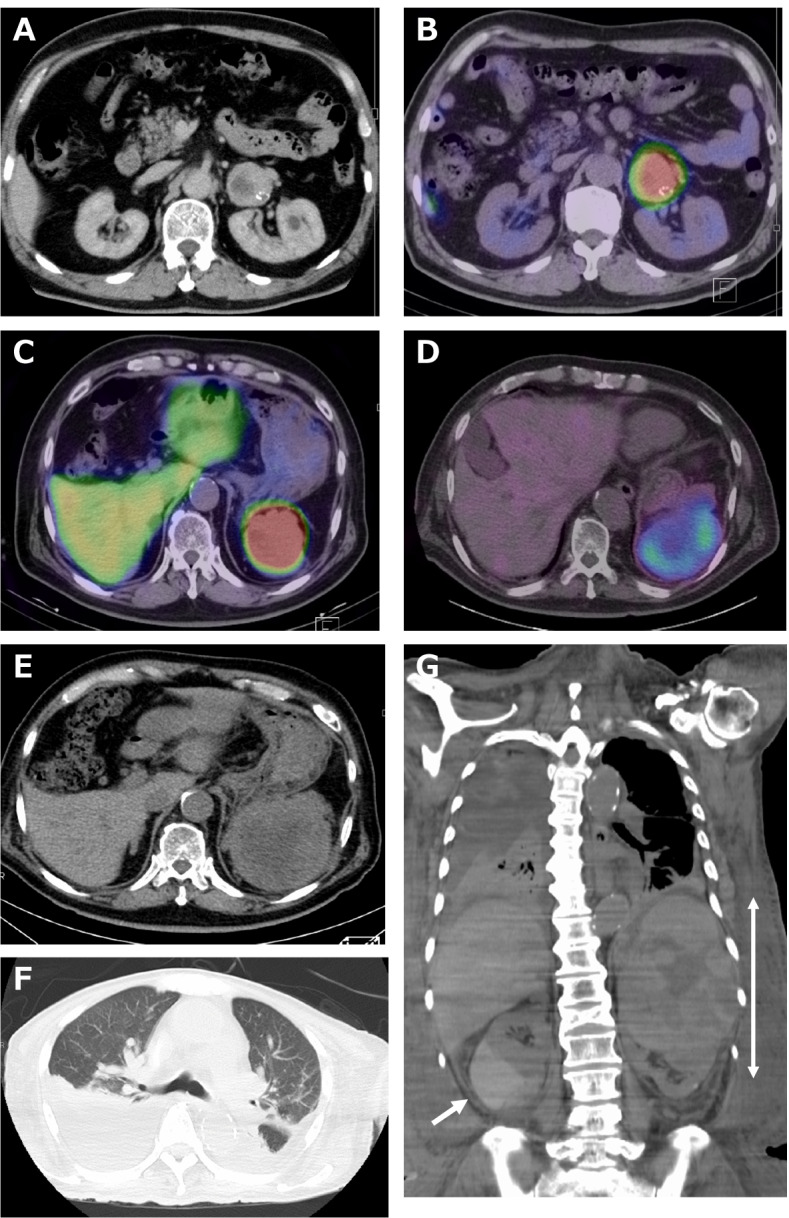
Representative images during the clinical course. A: CT image at the diagnosis of sole PCC. B: ^123^I-MIBG scintigraphy image at the diagnosis of sole PCC. C: ^123^I-MIBG scintigraphy image at the recurrence of PCC 7 years after surgery. D: SRS image at the diagnosis of recurrent PCC with simultaneous ACTH production. E–G: CT images at the terminal stage. Arrow in G indicates right adrenal subcortical bleeding. Double arrow in G indicates the maximum tumor diameter. MIBG, metaiodobenzylguanidine; SRS, somatostatin receptor scintigraphy
